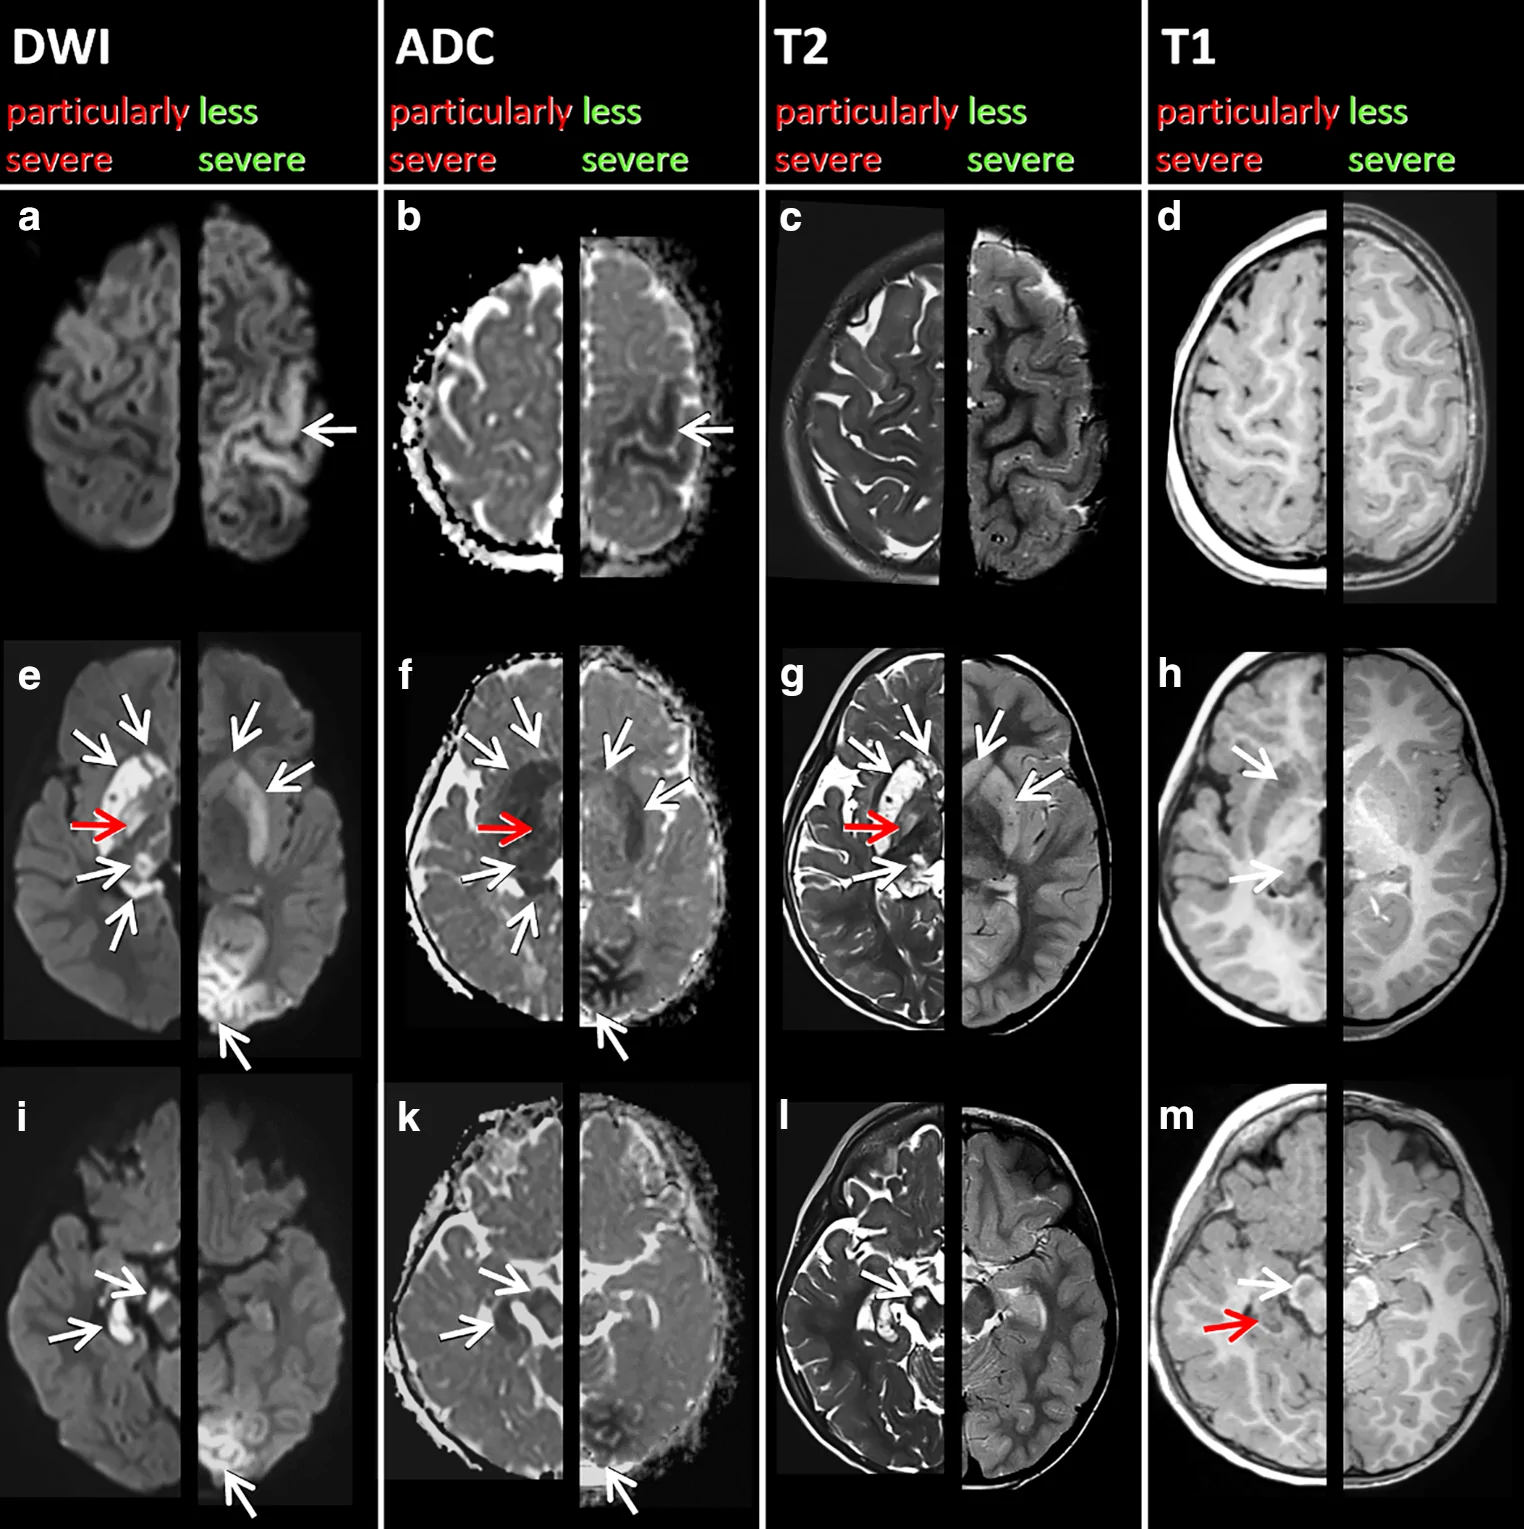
MRI obtained within 0–2 days after the anoxic event. Structures with abnormal signal intensities are marked with arrows (red/dark gray = predicting particularly severe outcome). Putamen and caudate nucleus (the “striatum”) were affected in almost all patients (arrows in e–h). Many patients showed also involvement of the thalamus (arrows in e–h), of primary cortical areas [i.e. of the primary sensorimotor cortex M1/S1 (arrows in a,b), of the primary visual cortex V1 (arrows in e,f,i,k), of the primary auditory cortex A1 (not shown)] and of the hippocampus (arrows in e–m)
